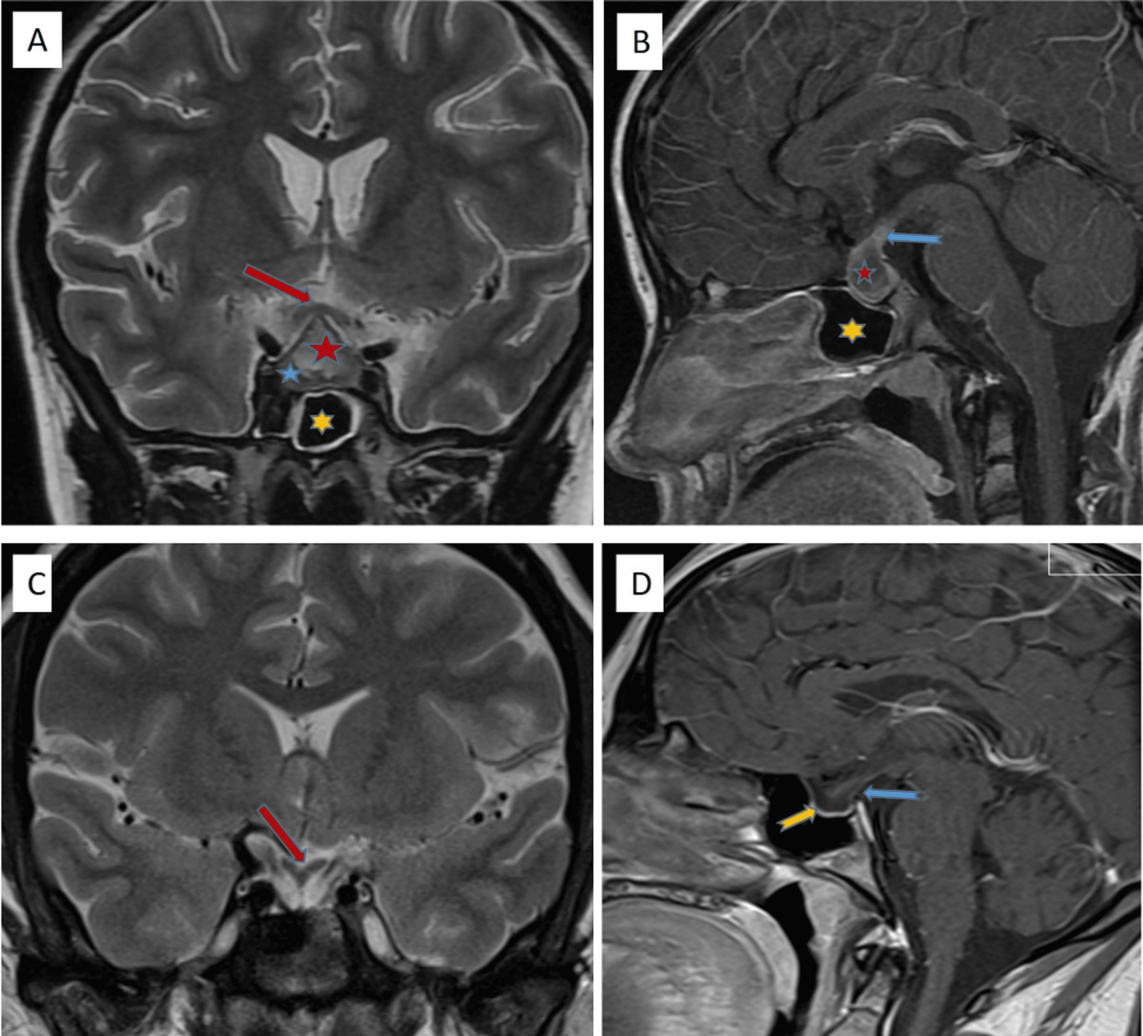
Brain MRI pre and post surgery A,B: Pre-surgery images; C,D: Post-surgery images A: T2 coronal image; inhomogeneous hyperintense, well-defined focal area (red star) in the anterior pituitary gland, and indentation on optic chiasm with cranial displacement (red arrow). PA. Normal glandular tissue is seen as a thinned area at caudal level (blue star). Mucosal thickenings are seen at walls of sphenoid sinus (yellow star). B: Post-contrast T1 sagittal image; thickened stalk (blue arrow) and peripherally irregular contrast enhancement is seen on PA (red star). Sphenoid sinus is represented by the yellow star. C: T2 coronal post-surgery image; caudally displacement/retraction of optic chiasm and stalk (red arrow) and significant volume loss at anterior pituitary gland (yellow arrow). D: Post-contrast T1 sagittal image; significant volume loss of anterior pituitary gland (yellow arrow) and thinned stalk (blue arrow). Empty sella findings. PA: Pituitary abscess
